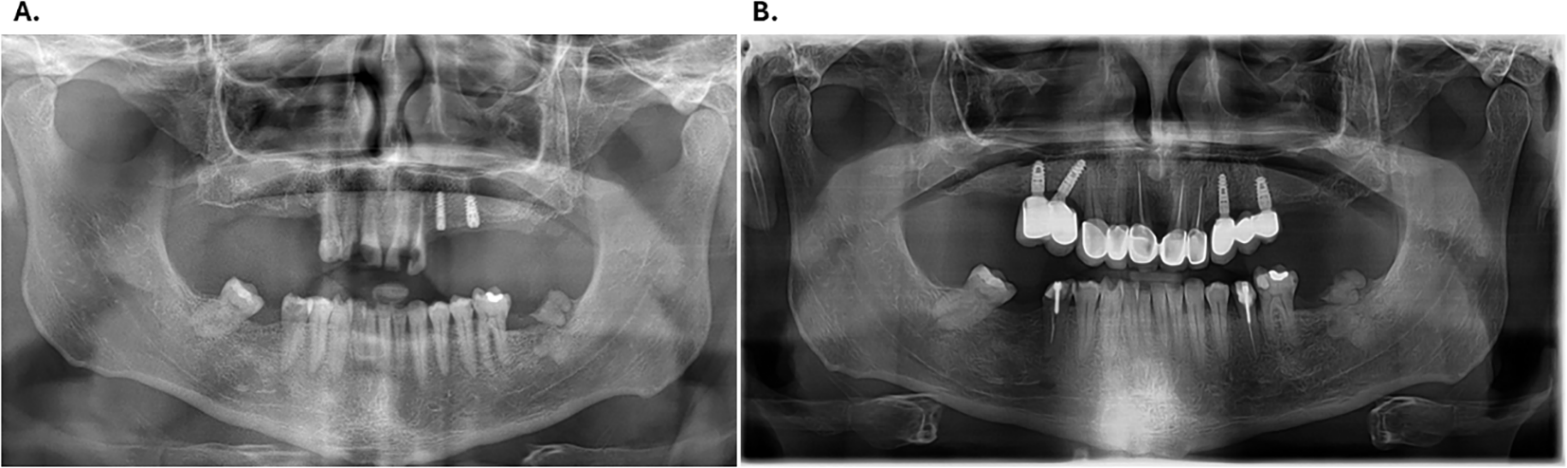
(A) Initial orthopantomogram; (B) Maxillary implants after the healing period.
